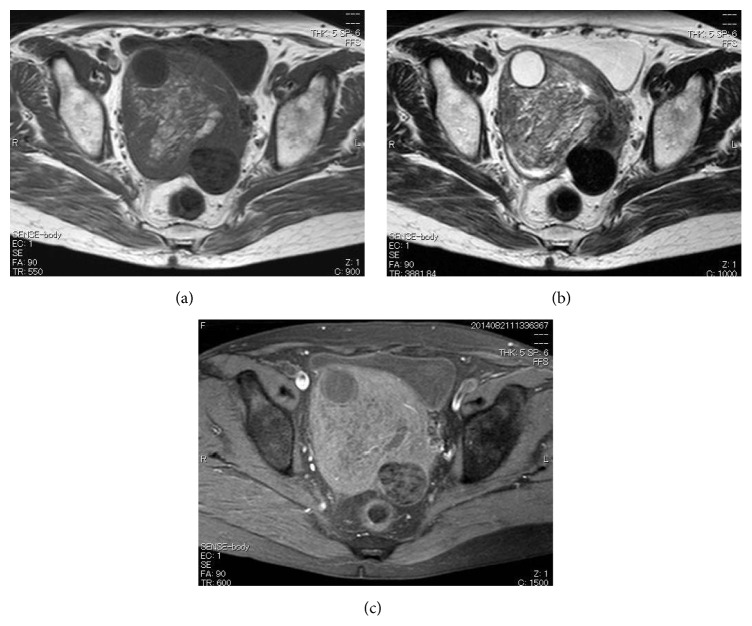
On referral to our institution, transverse magnetic resonance imaging sections through the pelvic tumor. (a) T1-, (b) T2-, and (c) fat-saturated T1-weighted images.
